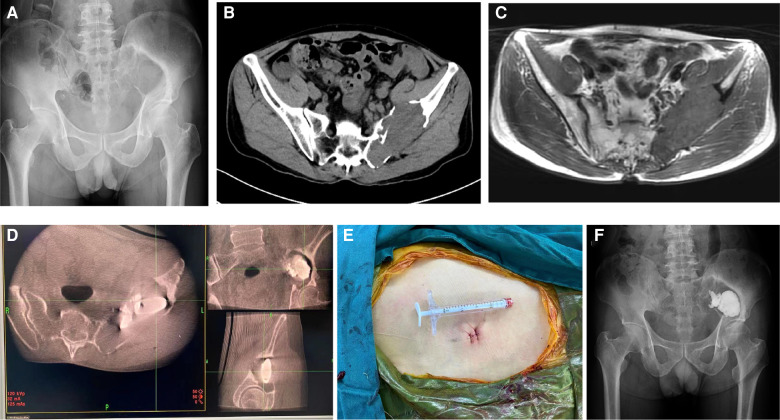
55-year-old man with metastatic renal cell carcinoma and a painful pelvic metastasis. (A) Preoperative pelvic x-ray. (B,C) Preoperative pelvic CT and MRI scans. (D) Intraoperative O-arm 3D image during puncture and cementoplasty. (E) Skin incision. (F) Postoperative pelvic x-ray 6 months after the procedure.
